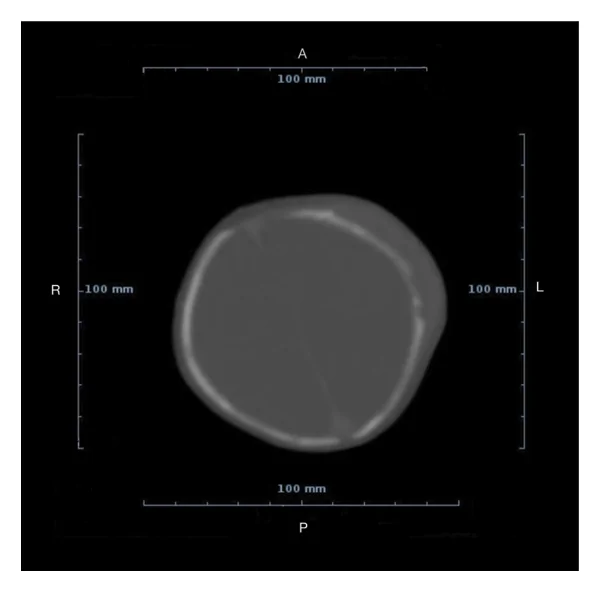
CT head with contrast (bone window); the green arrow shows the residual infected subgaleal hematoma, and the blue arrow shows the irregular thickness and reduced density of the underlying bone representing the osteomyelitis.
